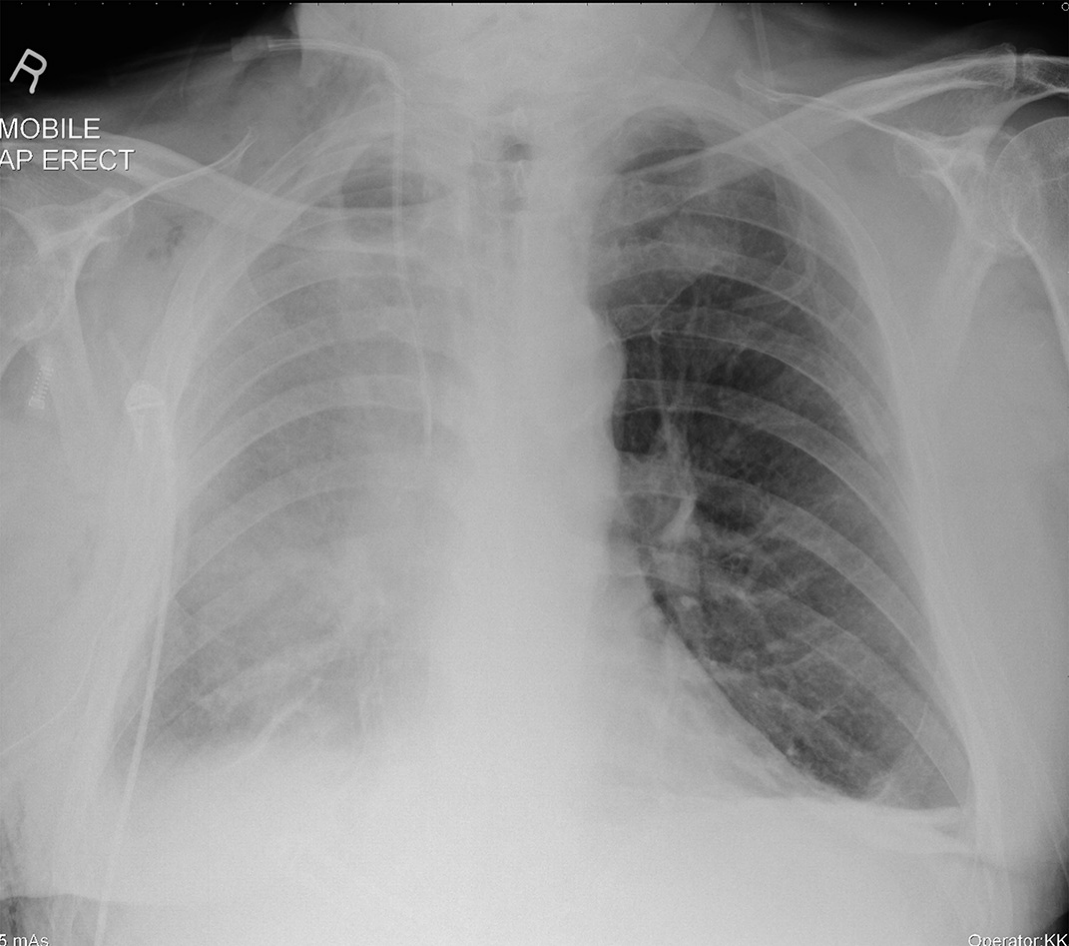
Chest radiograph on post-operative day 3 confirms further deterioration, with more extensive opacification projected over most of the hemithorax but sparing the costophrenic angle and apex. AP, anteroposterior.
